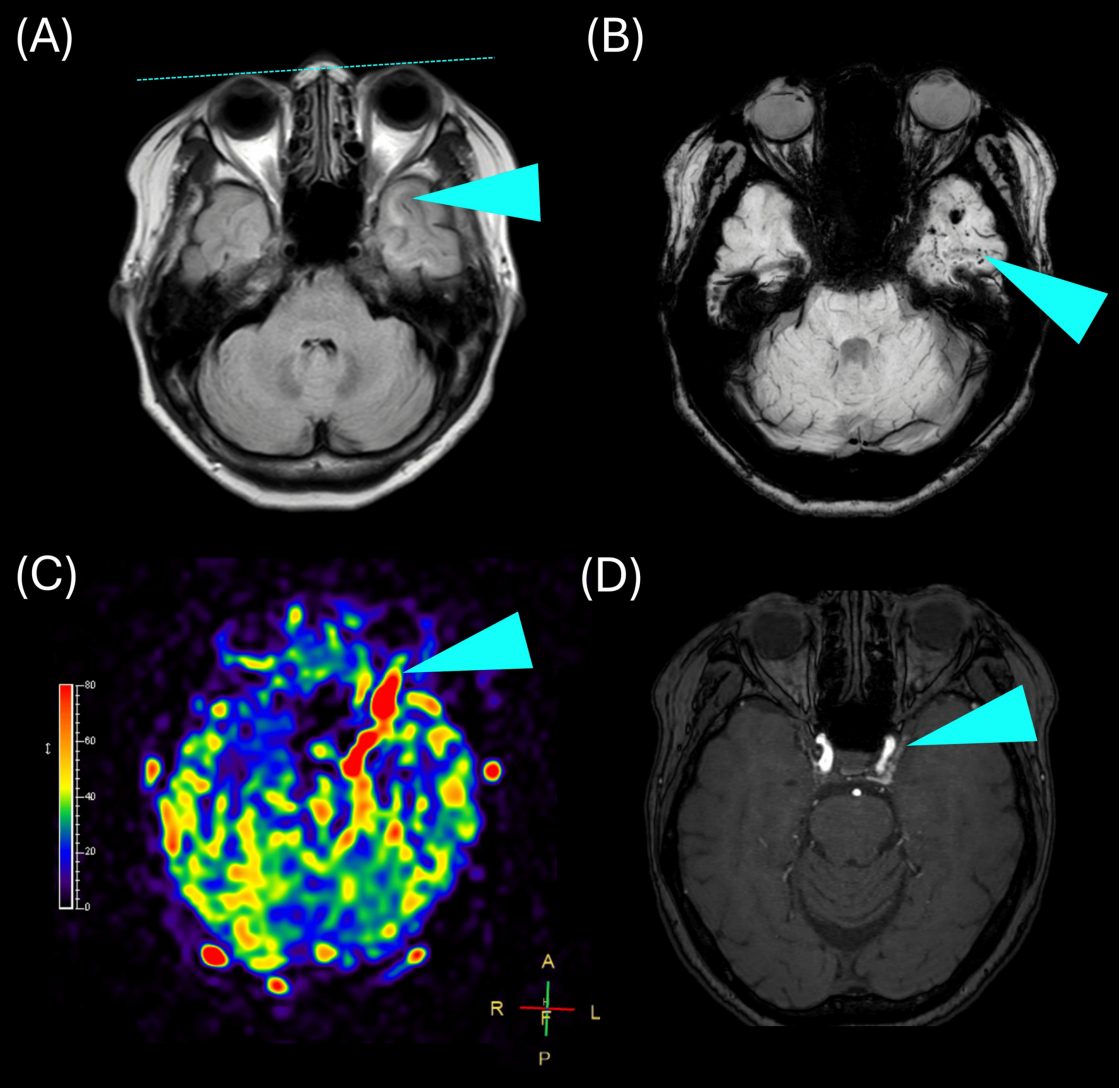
MRI and MRA findings of the left CS-DAVF (A) FLAIR image showing subcortical white matter edema in the left cerebral hemisphere. (B) SWI demonstrating numerous punctate microhemorrhages in the left subcortical white matter. (C) ASL image indicating increased blood flow extending from the left orbit to the parasellar region. (D) MRA clearly visualizing the enlarged left cavernous sinus with prominent internal flow voids. MRI: magnetic resonance imaging, MRA: magnetic resonance angiography, CS-DAVF: cavernous sinus dural arteriovenous fistula, FLAIR: fluid-attenuated inversion recovery, SWI: susceptibility-weighted imaging, ASL: arterial spin labeling
